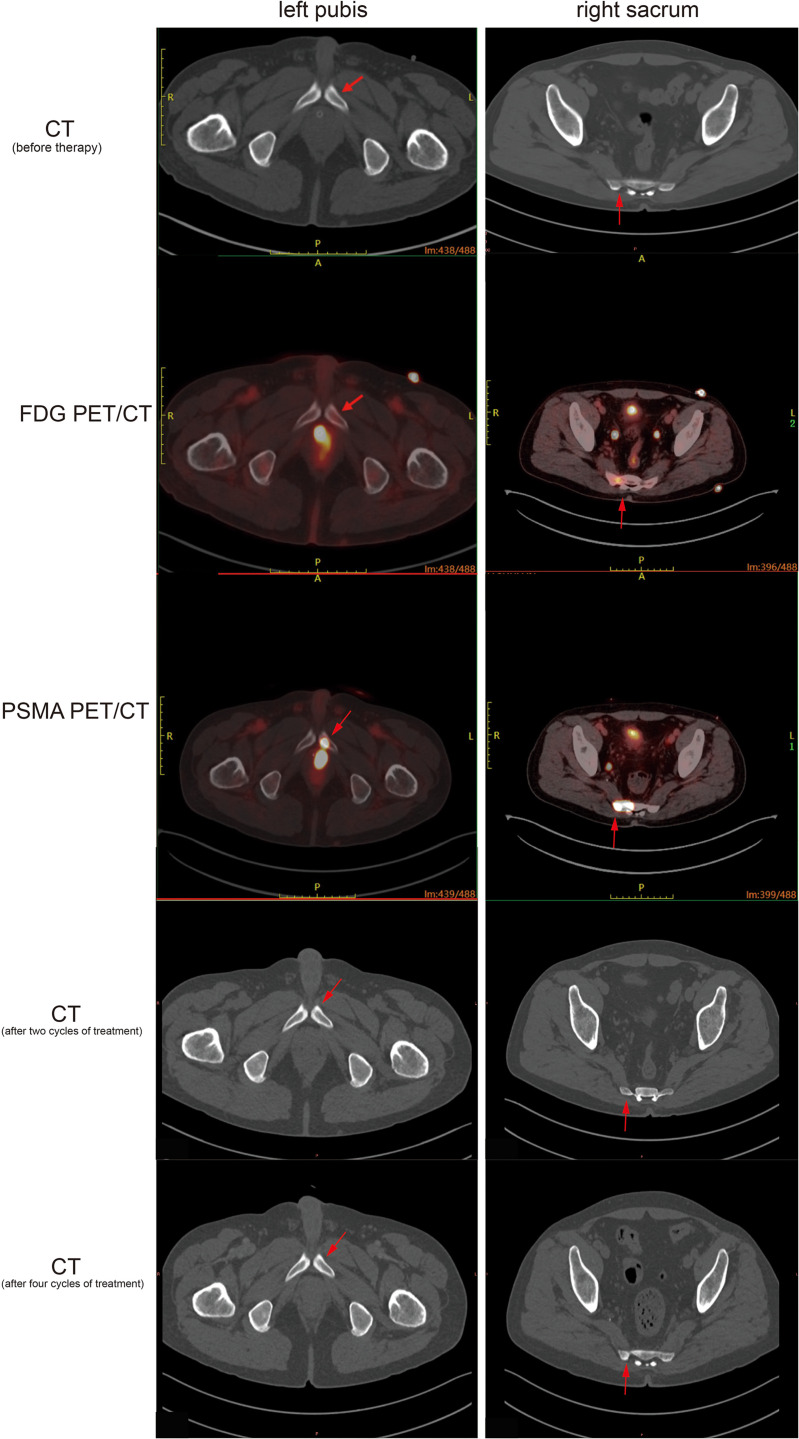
Representative PET/CT and CT images demonstrating PCa metastases in the left pubis and right sacrum with markedly elevated PSMA uptake (SUVmax 24.3 and 41.3, respectively) and only mildly increased FDG uptake (SUVmax 2.0 and 4.7, respectively), while no obviously identifiable metastatic lesions were shown on the corresponding CT images, which remained stable throughout the whole treatment period.
